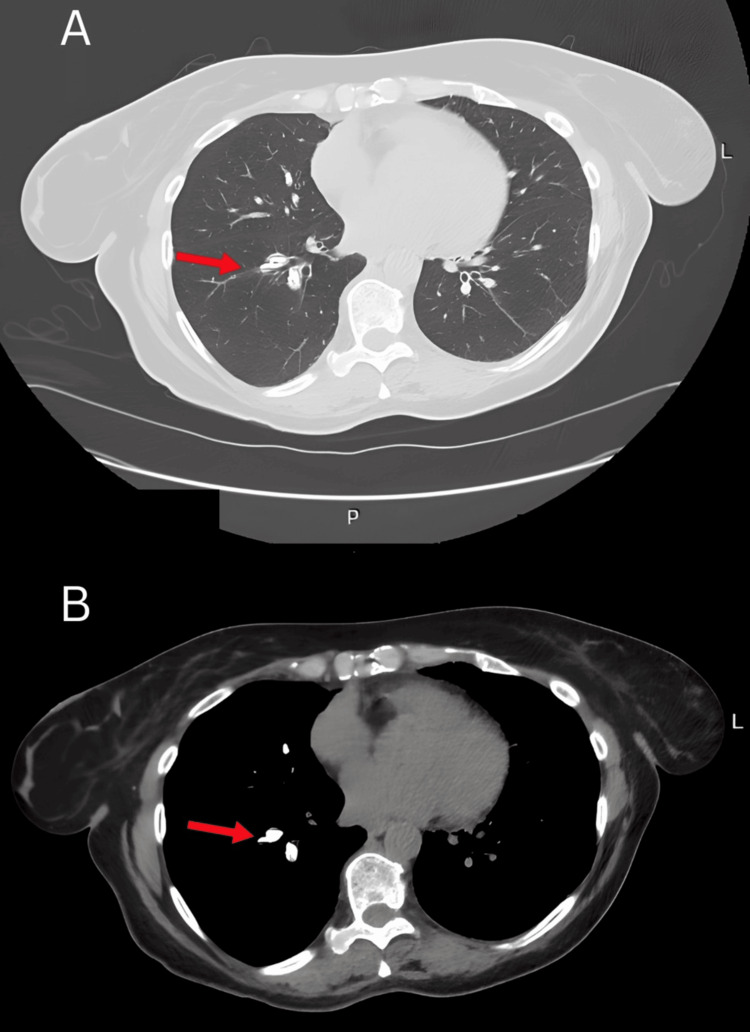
Thorax CT scan. A: lung window; B: mediastinal window projection. The PMMA presence in pulmonary vessels is depicted with red arrows as hyperdense opacities circumferential to the pulmonary vessels. PMMA: polymethyl methacrylate
